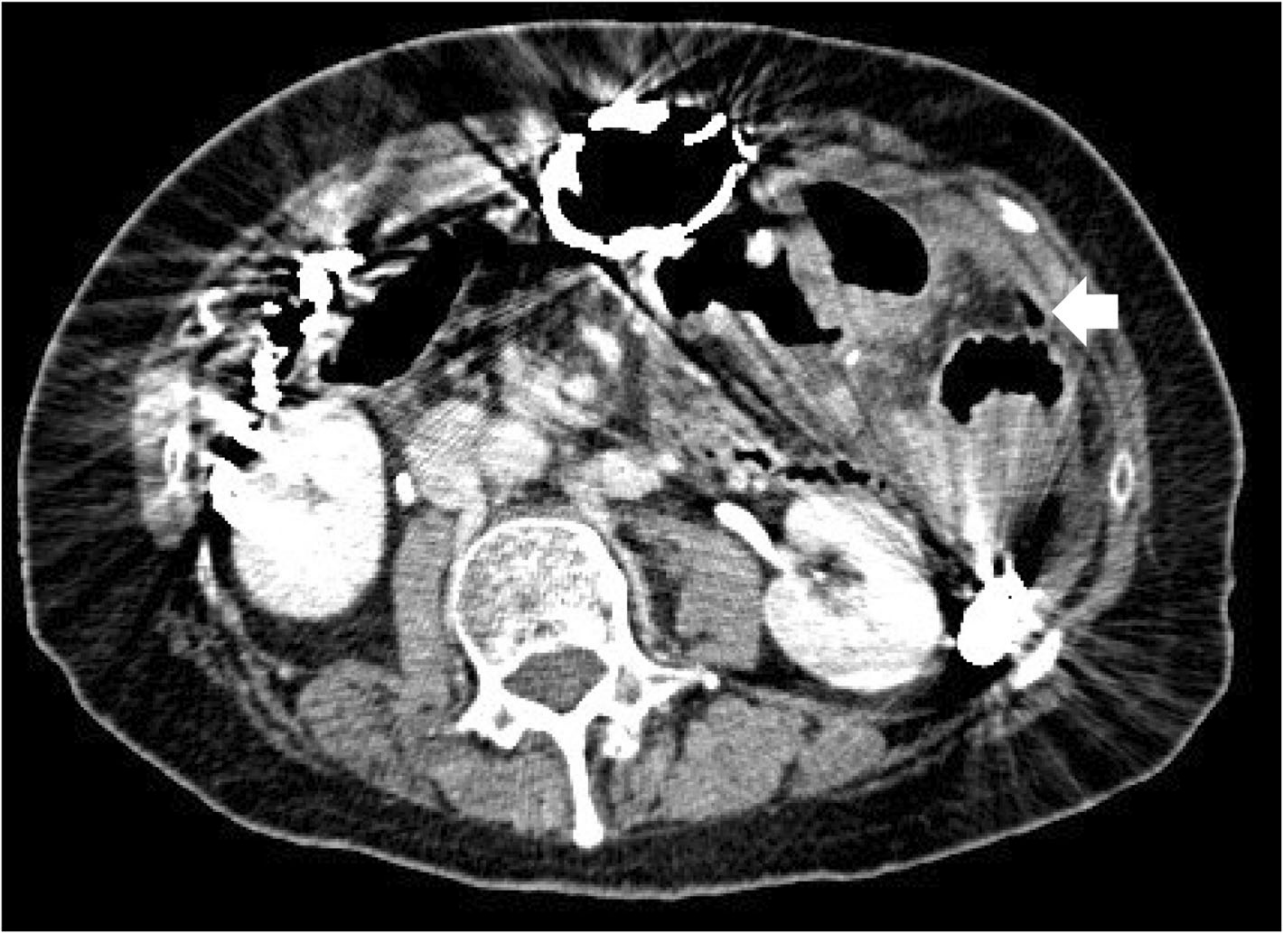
Computed tomography revealed free intraabdominal air near the anastomosis site (arrow)
